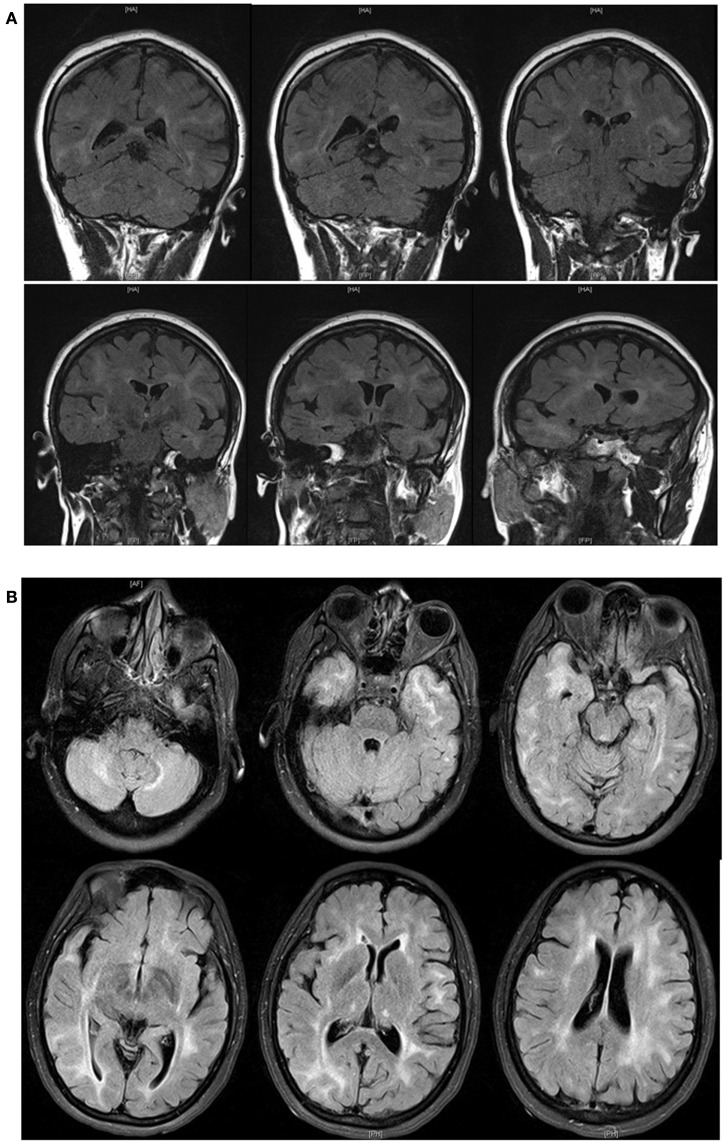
Fluid attenuated inversion recovery (FLAIR) MRI [(A),coronal; (B), axial] demonstrated showing diffuse abnormalities involving the periventricular and subcortical white matter of both cerebral hemispheres, areas of punctate hyperintensities on both thalami, and foci of hyperintense signal on both cerebellar hemispheres.
